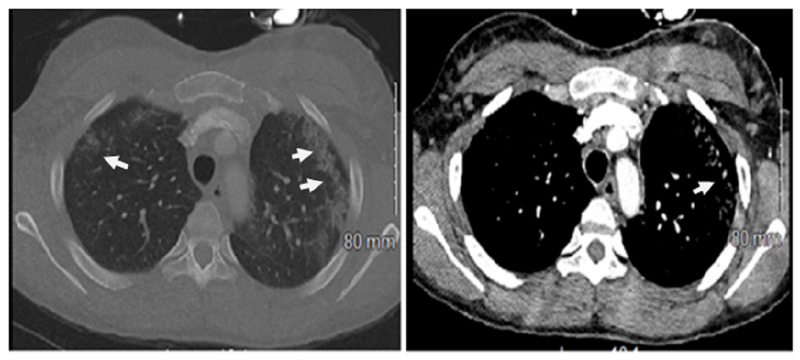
Computed tomography of the chest showing bilateral interstitial opacities, ground-glass opacities, and bronchiectasis
